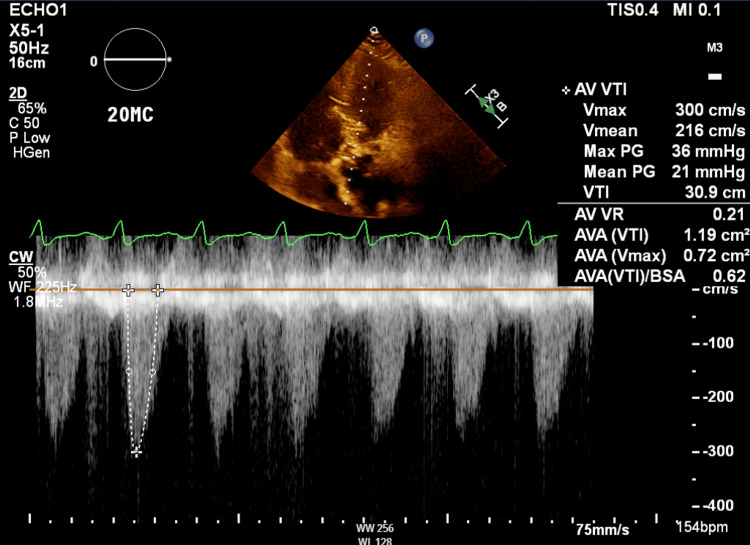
Preprocedural continuous wave Doppler of the aortic valve during dobutamine stress echocardiography at the apical four-chamber view, with findings consistent with severe valvular aortic stenosis
